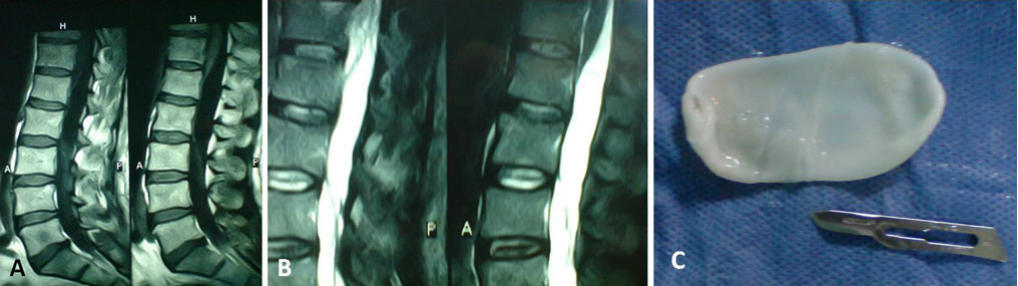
a, b Magnetic resonance imaging of the lumbar spine showed a cystic lesion compressing the thecal sac, involving the L1–L2. c A classic milky-white cyst wall was excised
